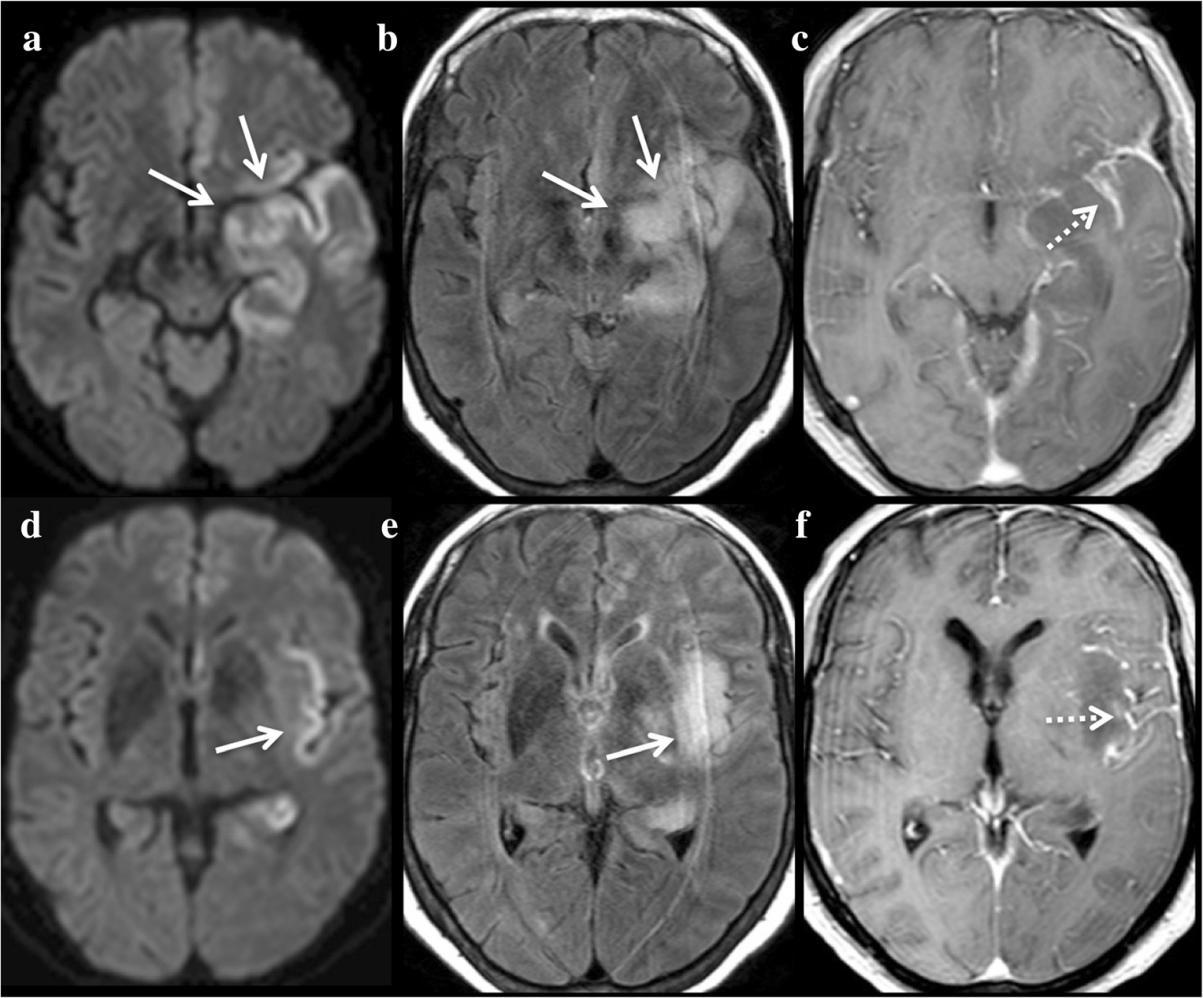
A 63-year-old woman with herpes encephalitis presenting with aphasia, cephalalgia and hyperthermia. DWI and FLAIR show left temporal and insular hyperintensities involving the uncus (a, b, d, e, arrows). Contrast-enhanced T1-WI shows leptomeningeal enhancement related to the associated meningitis (c, f, dotted arrows)
